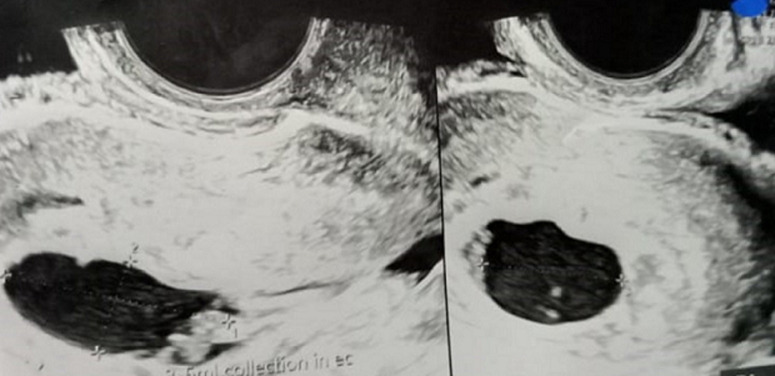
trans abdominal sonography showing 3-5 cc of collection in endometrial cavity
